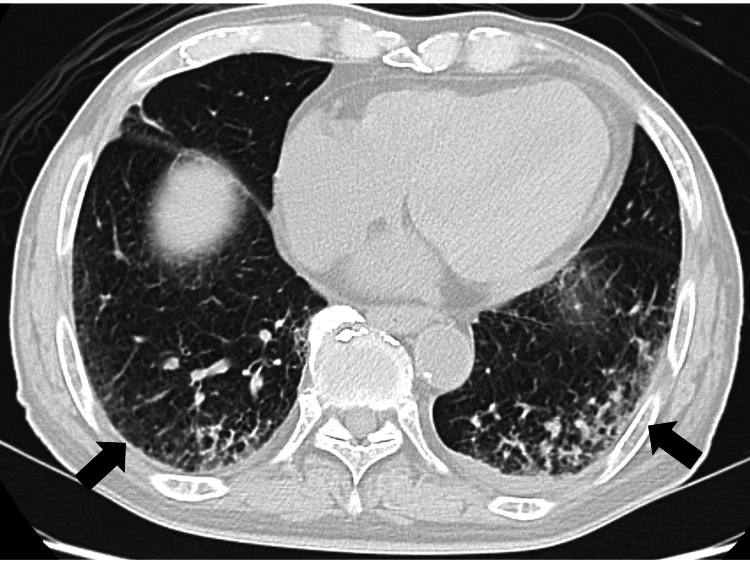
Chest computed tomography image showing infiltration in the bilateral lower lobes of the lungs.
